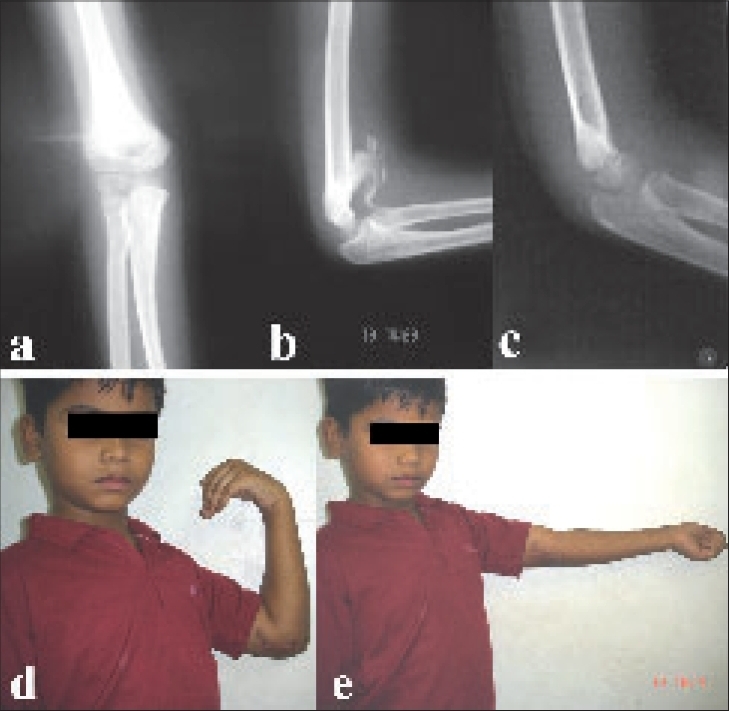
Preoperative anteroposterior (a) and lateral radiographs (b) of right elbow in 13 Year old male showing anterior heterotopic bone causing limitation of flexion. Postoperative lateral radiographs (c) following arthrolysis and removal of anterior bony block. Clinical photographs (d and e) showing a good range of flexion and extension at follow up.
